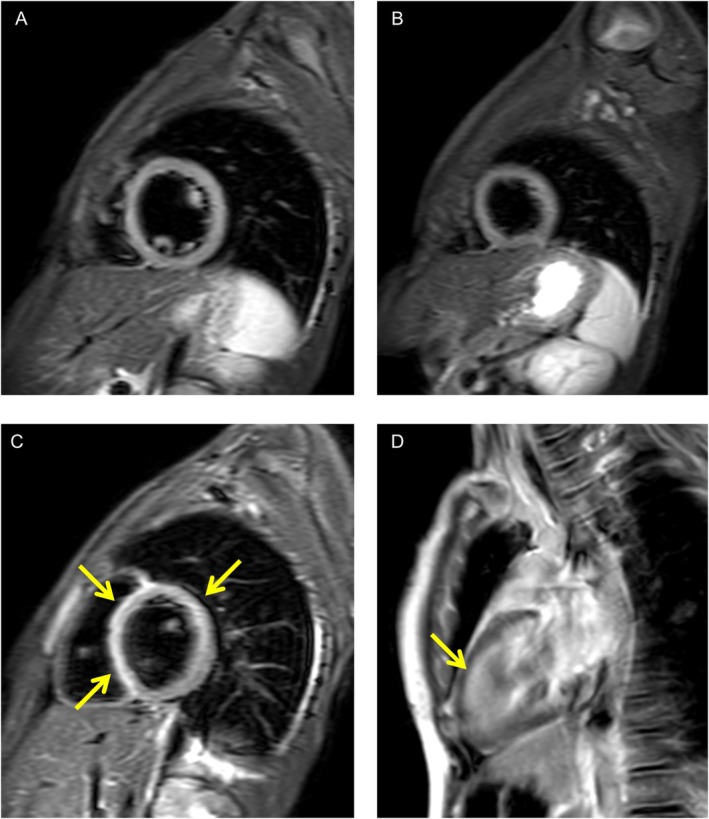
Cardiac magnetic resonance imaging in this patient. (A) Dark Blood T2 Short Inversion Time Reversal (STIR) image. No myocardial edema is observed. (B) Cine does not show an obvious localized wall motion abnormality. (C, D) Late gadolinium enhancement (LGE) imaging shows localized hyperintense signal within the myocardium. Yellow arrows indicate the enhancement.
